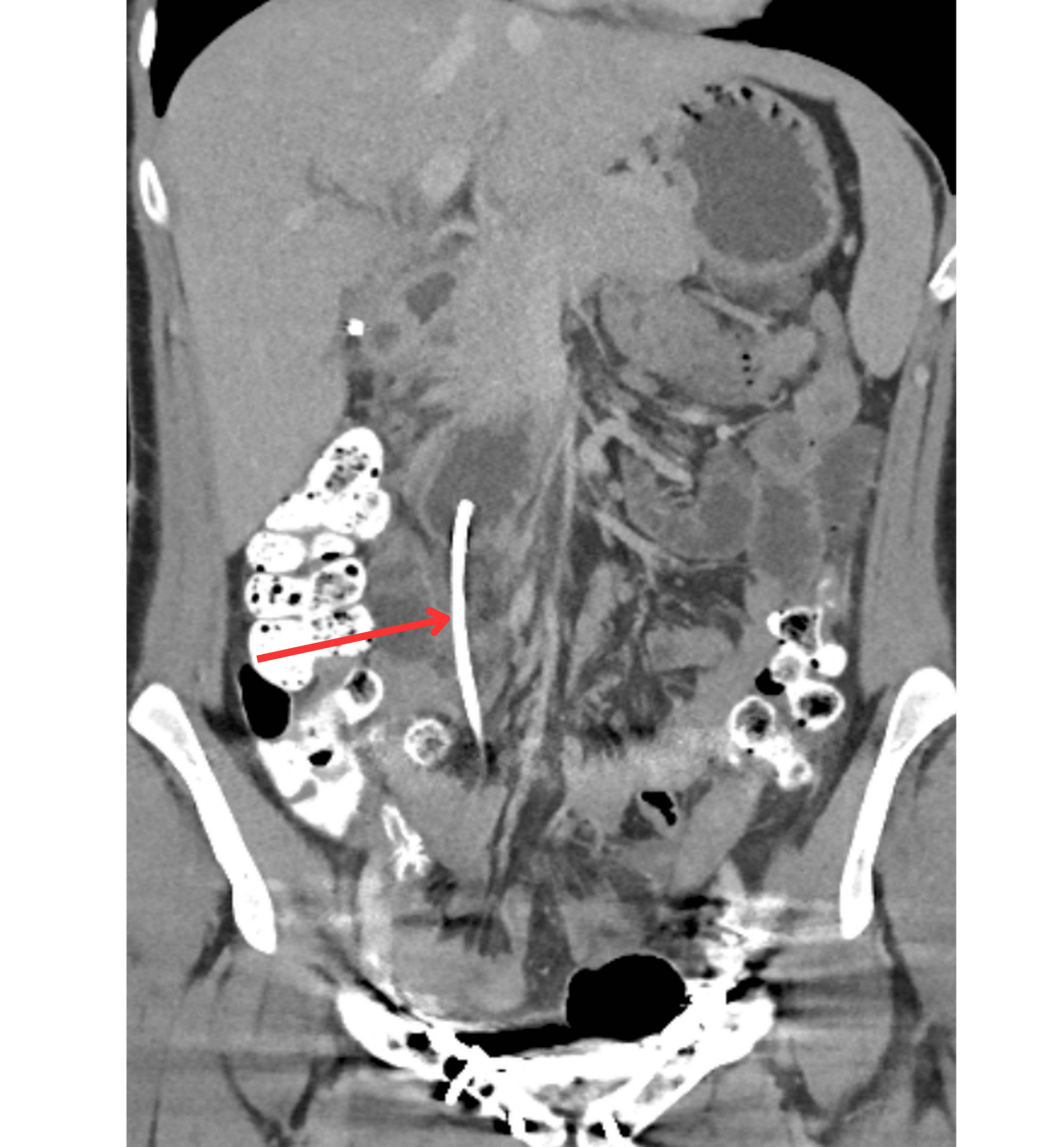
CT of the abdomen and pelvis showing approximately 17 mm of the stent lying within the lumen of the duodenum. The remainder of the stent protruded through the duodenal wall (approximately 10 cm long).
